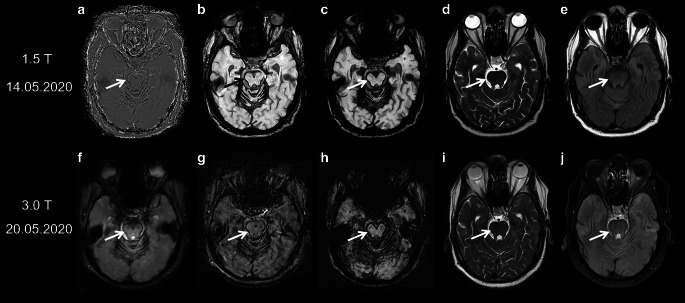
Diagnostic value of different MRI sequences in the detection of superficial siderosis (SS). A 61-year-old man with SS due to ongoing hemorrhage from a melanoma metastasis in the right frontal cortex. In T2*-GRE (f) and susceptibility-weighted imaging (SWI) (b,g), SS is revealed by dark rims on the surface of affected structures, e.g., the mesencephalon (*arrow*), with SWI being more sensitive. Minimum intensity projections (mIPs) of SW images (c,h) further enhance the conspicuousness of SS. In addition, filtered phase image of SWI (a) can be used to distinguish paramagnetic (hemorrhage/iron, *dark* here) from diamagnetic substances (calcification, *bright* here), as they have opposite signal intensities. In general, susceptibility effects are more pronounced on images acquired at 3 T (f–j) than on images acquired at 1.5 T (a–e). Whereas at 3 T a hypointense rim around the mesencephalon is seen in T2WI and fluid attenuated inversion recovery (FLAIR) images (i,j, *arrow*), SS is almost undetectable at 1.5 T in T2WI and FLAIR (d,e, *arrow*). a–e 1.5 T (Achieva dStream, Philips); a–c SWI, TR/α 52 ms/20°, 4 echoes TE1 = 12 ms, ∆TE = 11 ms; d T2, TR/TE/α = 5762 ms/110 ms/90°; e FLAIR, TR/TE/TI/α = 11000 ms/140 ms/2800 ms/90°; f–j 3 T (Skyra fit, Siemens); f T_2_*-GRE, TR/TE/α = 631 ms/20 ms/20°; g, h SWI, TR/TE/α = 27 ms/20 ms/15°; i T2, TR/TE/α = 4980 ms/92 ms/150°, j FLAIR: TR/TE/TI/α = 8500 ms/81 ms/2440 ms/150°. *TR* repetition time, *TE* echo time, *TI* inversion time, *α* flip angle
